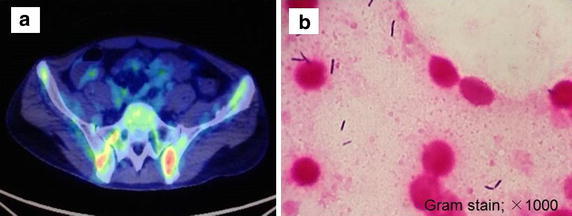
Diagnostic approach for a 32-year-old male with *Clostridium innocuum* osteomyelitis. a (18F)-Fluorodeoxyglucose positron emission tomography (FDG-PET) revealed marked uptake of FDG in the sacroiliac joint and iliac bone. b Bone marrow biopsy from iliac crest confirmed *C. innocuum* infection (Grambiopsy conf1000).
